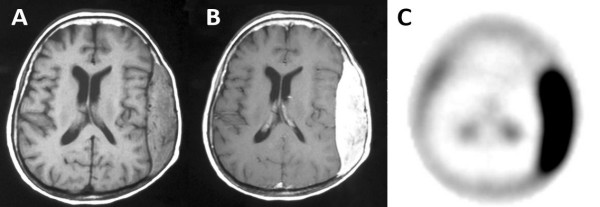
A. Axial T1-weighted MR image revealing an isointense signal mass in the left temporoparietal region. B. Marked enhancment after contrast administration. C. Reconstructed axial SPECT slice revealing significant ^99m^Tc-Tetrofosmin uptake in the left temporoparietal region.
